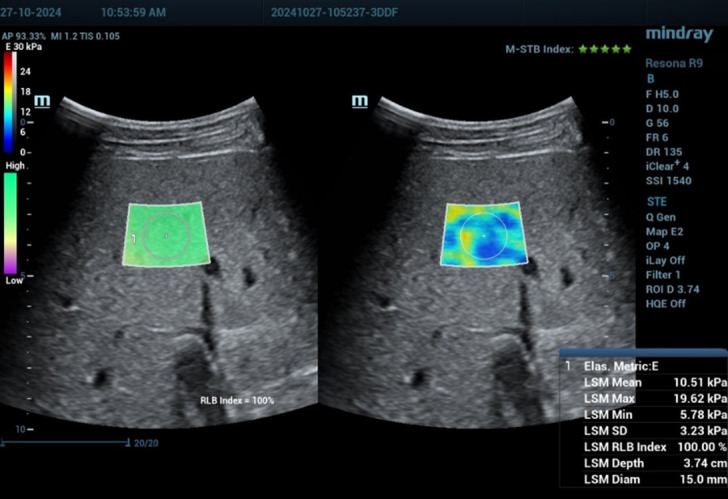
Asymptomatic 24 years old male patient who is addict on crystal methamphetamine (urine test +Ve) for 3 years with elevated liver enzymes. Liver stiffness value obtained by two-dimensional shear wave elastography technique (STE technique by resona R9 ultrasound machine of mindray medical systems) with the mean was 10.5 kPa indicating a significant liver fibrosis with respect to manufacturer reliability and stability index and measurement IQR/median <10. With standardized box positioning.
